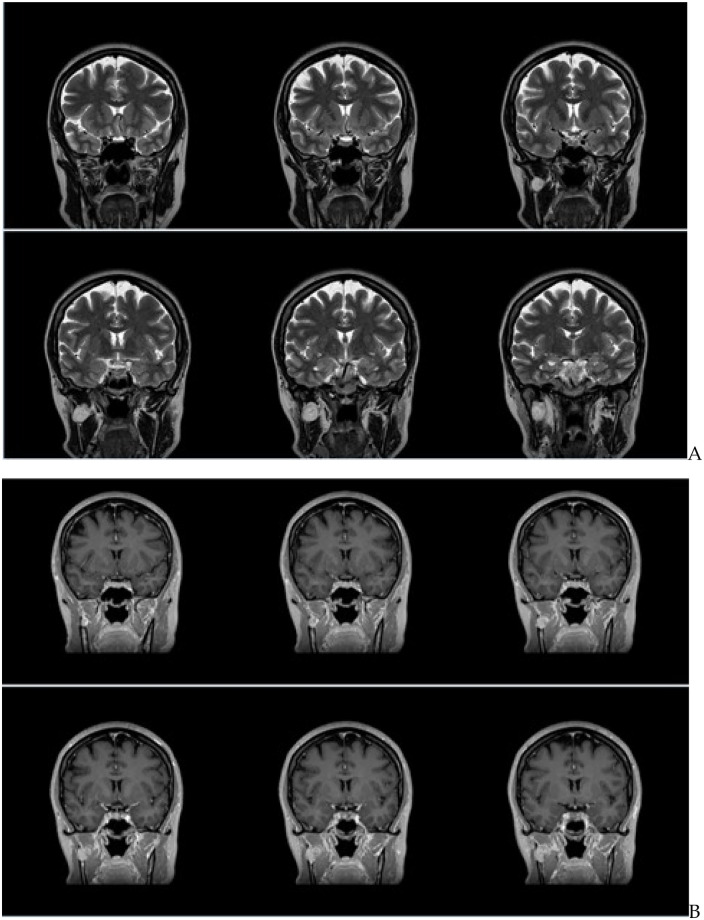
(A) MR T2-weighted. (B) MR T1-weighted.
